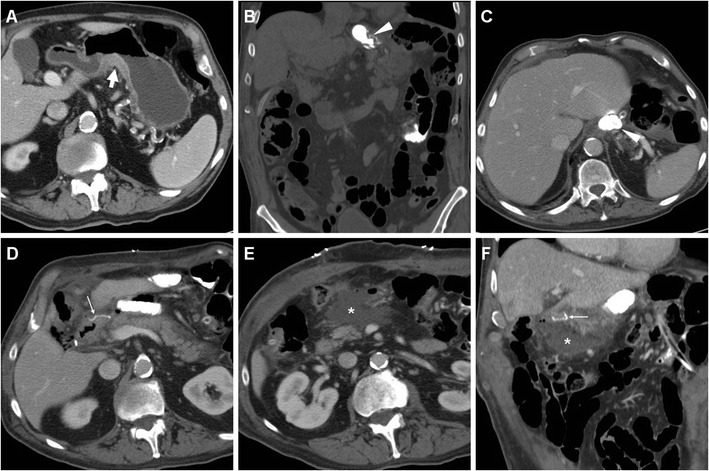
An 81-year-old male with T3 N2 cancer of the lesser gastric curvature (thick arrow) as preoperatively depicted by CT with oral water distension (A) underwent total gastrectomy with Roux-en-Y reconstruction. On 9th POD CT was performed because of bile from drainage: unenhanced (B) and post contrast (C-F) scans with oral contrast showed normally opacified and patent EJS (arrowheads) without evidence of anastomotic leak (note tip of surgical drainage abutting the EJS in C). Abutting the stapled DS (thin arrows) a non-capsulated fluid collection (*) corresponded to DS leakage which was conservatively managed
